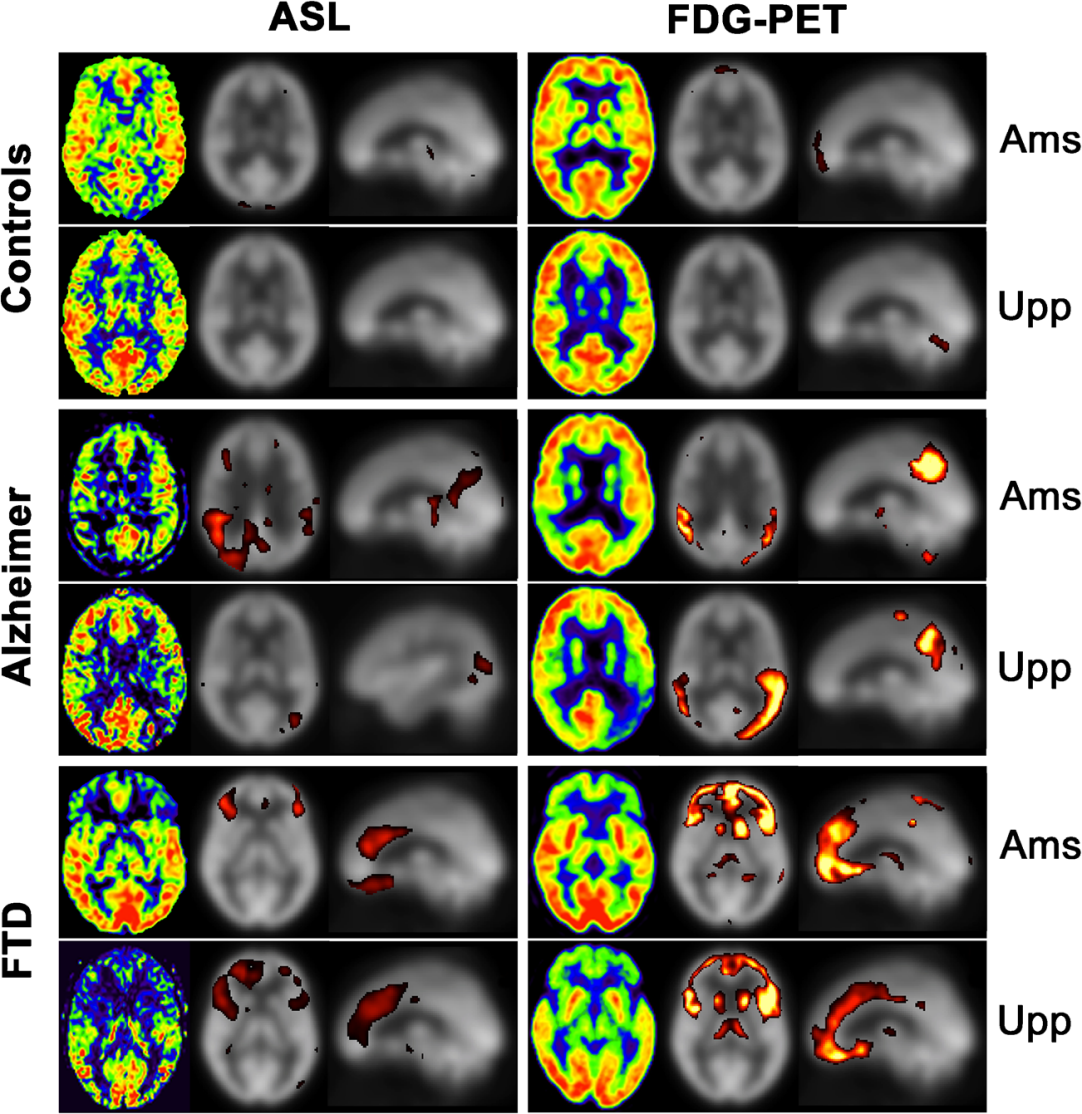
Representative subjects. Sample images from one control, one AD patient and one FTD patient from each site. Images are shown pairwise from the separate sites. ASL- and FDG-based images are shown in separate columns. The leftmost image in each box is an axial slice from the original image shown in a manually windowed Osirix rainbow scale. The middle and rightmost images in each box are axial and sagittal Z-maps created from the respective modality, with thresholded overlays shown in a dichromatic colour scale with the default lower and upper Z-score thresholds of 2.0 (red) and 6.0 (yellow). *AD* Alzheimer’s disease, *FTD* frontotemporal dementia, *ASL* arterial spin labeling, *Ams* Amsterdam, *Upp* Uppsala
